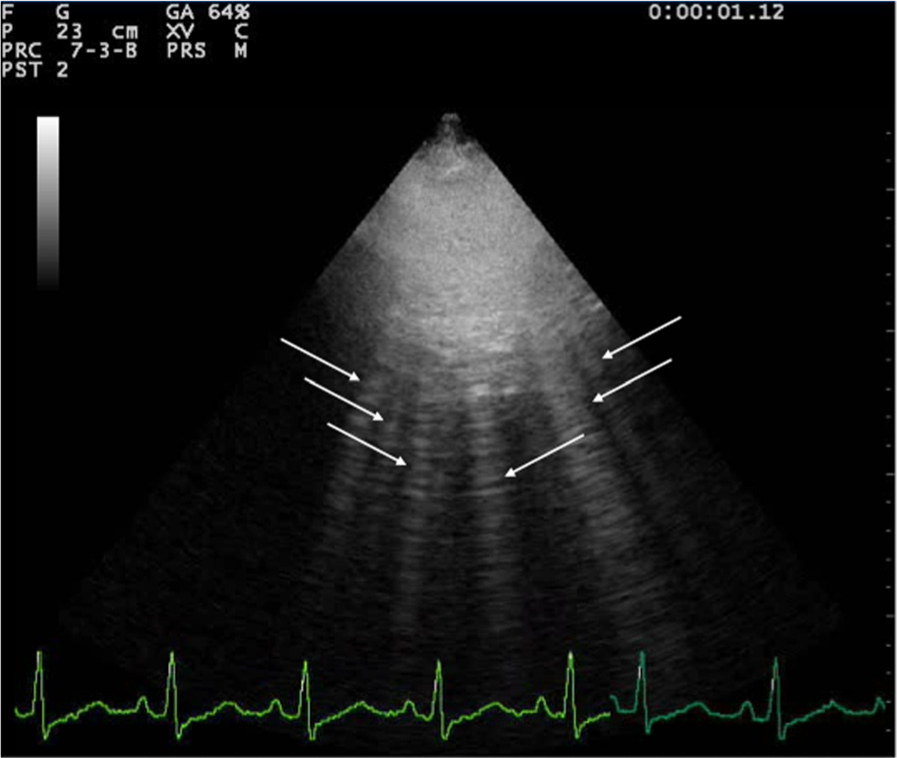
Sonographic appearance of multiple B-lines (indicated by the white arrows).
